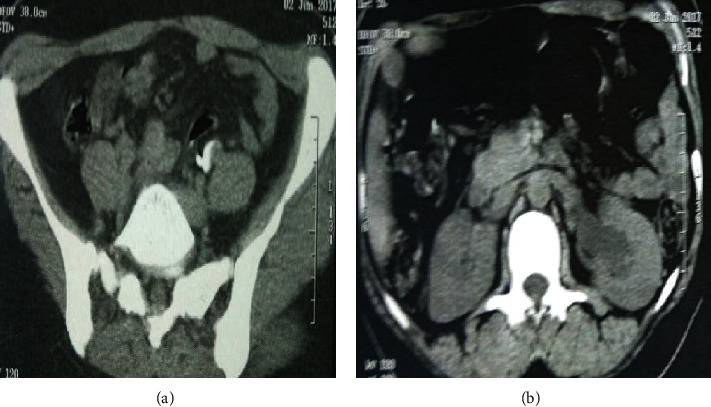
CT image (cross section). (a) CT image showing the retroperitoneal location with left ureteral compression. (b) CT image showing left ureterohydronephrosis secondary to ureteral compression by the IUD.
